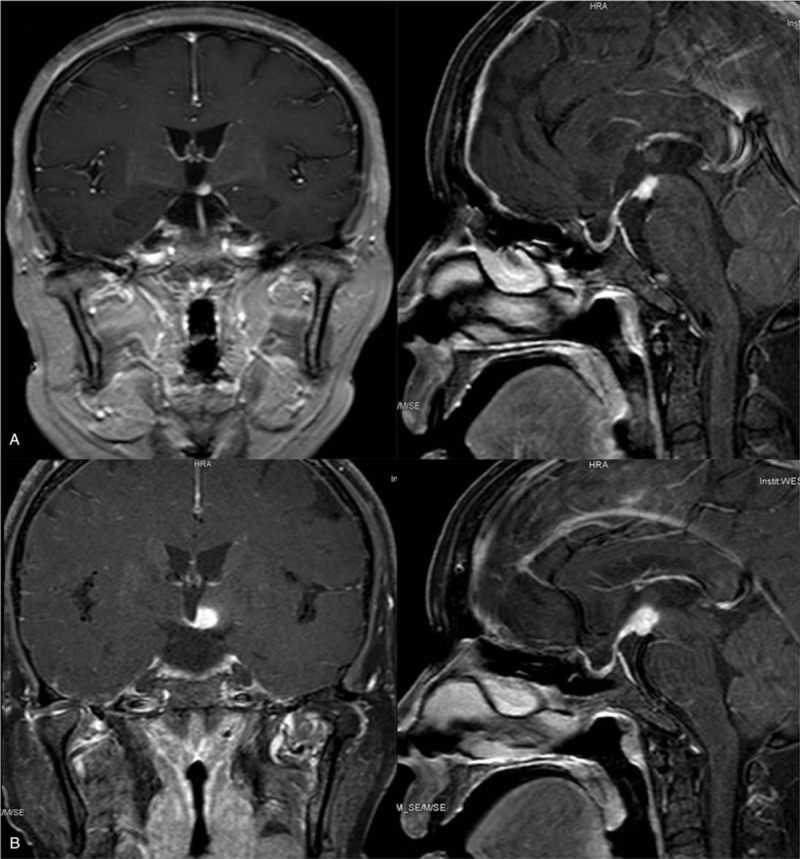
Enhanced MRI of sella region in 2016 (A) and 2017 (B). A) Enhanced MRI in 2016 showed bone flap of the right frontal bone with adjacent meninges thicking, and long T1 and T2 signal in bilateral centrum semiovale. Encephalocoele and cisterns showed no evident abnormalities. There was an enhanced nodule (0.8 cm) in the left hypothalamus. B) Enhanced MRI in 2017 showed enhanced nodule (1.4 cm) in the left hypothalamus and pituitary stalk thicking. Long T1 and T2 signal located in bilateral centrum semiovale. Encephalocoele and cisterns were normal.
